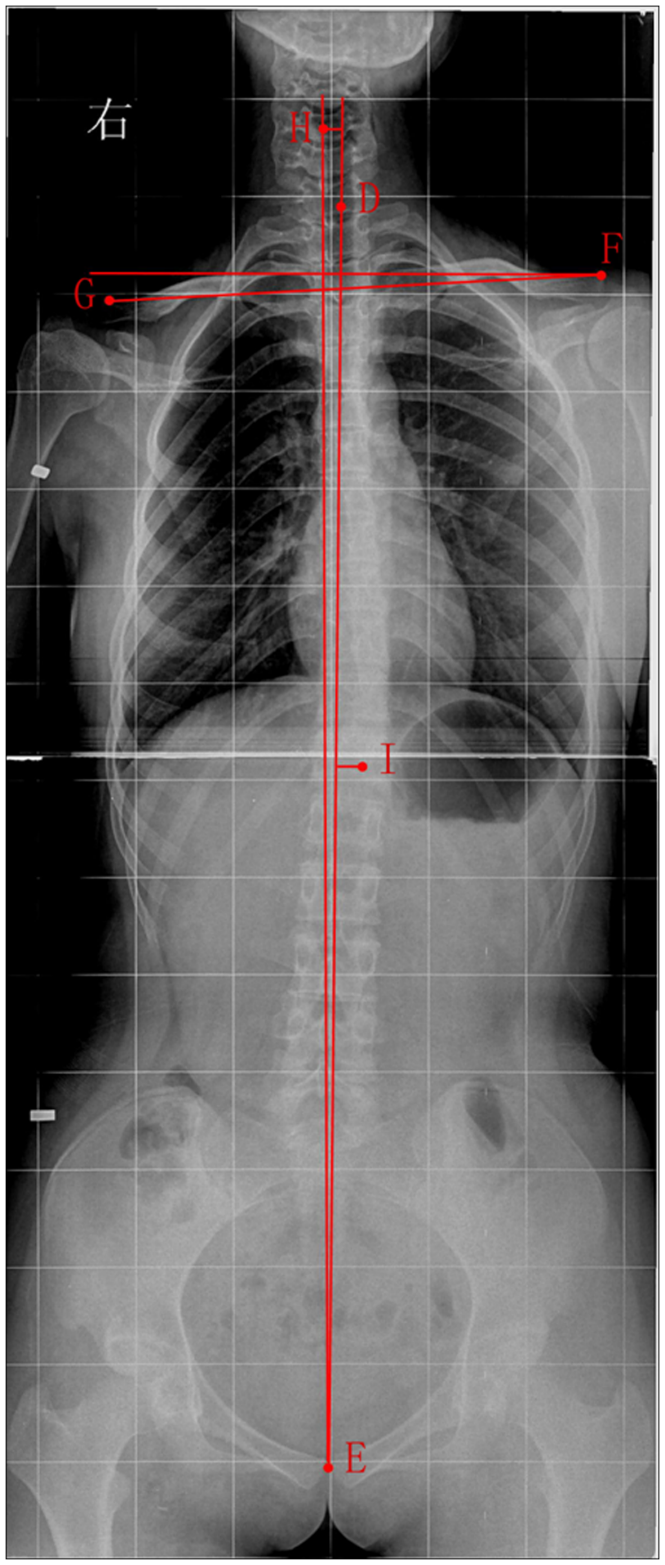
Standing anteroposterior X-ray film of the full-length spine. The midpoint of the 7th cervical vertebra was designated as point D, the midpoint of pubic symphysis point E, and the shoulder peaks as points F and G. In the cervical and thoracic vertebrae, the midpoints of the upper most scoliotic vertebra were designated as points H and I.
